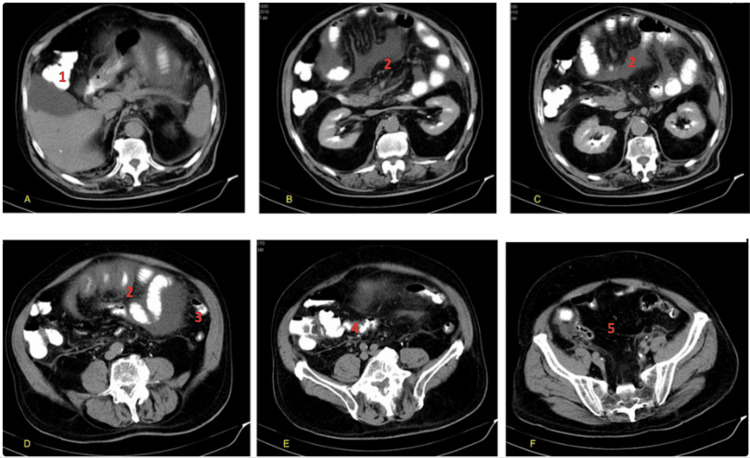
CT scan showcasing SEP and persistent descending mesocolon (A-F). SEP: sclerosing encapsulating peritonitis The images show (1) right colic flexure, (2) thickened and dilated small bowel loops located in the epigastrium, (3) splenic flexure, and (4) transverse colon located below the small bowel and (5) pelvis. No small bowel loops were found in the iliac fossa (only cecum is visible).
